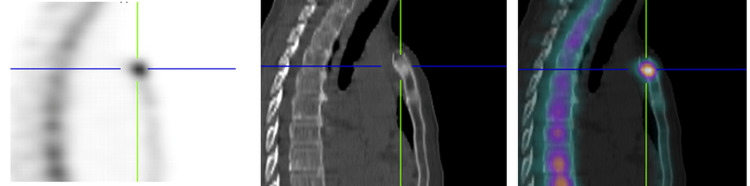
SPECT-CT imaging revealed an increased uptake within the lesion and a sclerotic appearance without any evidence of cortical destruction in the manubrium sterni. SPECT-CT: single-photon emission computed tomography
